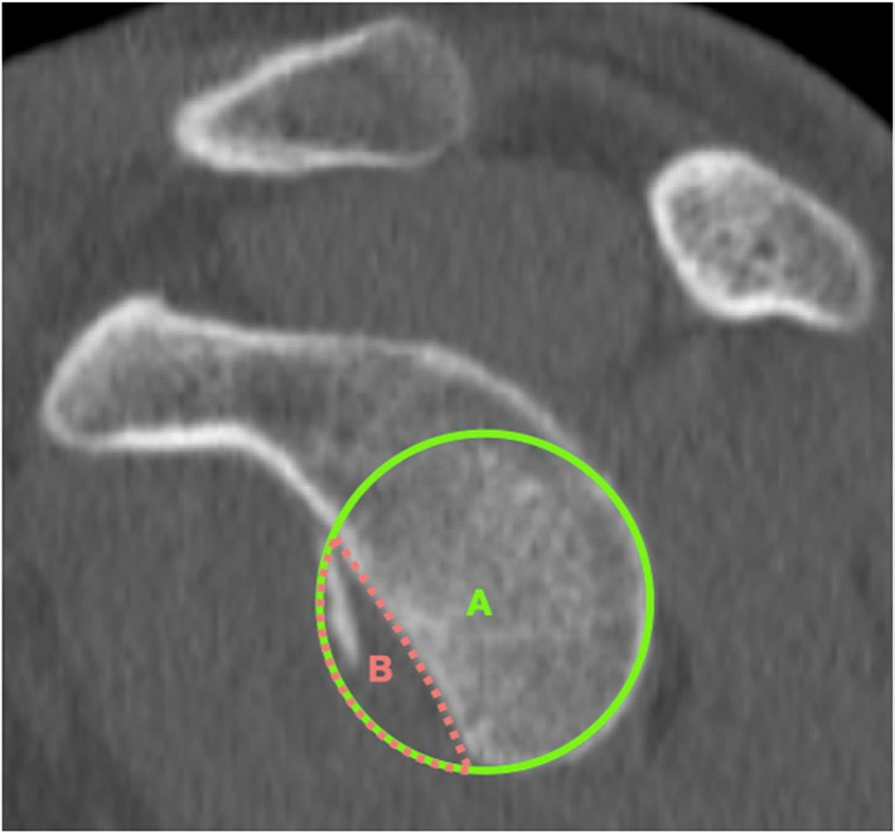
a CT en-face image of the left shoulder of a subject with anterior shoulder instability and apparent anterior glenoid bone loss. The glenoid bone defect is measured using the PICO method. A best-fit circle is drawn around the posteroinferior cortical margin of the glenoid. The defect is manually drawn and measured using the area tool
